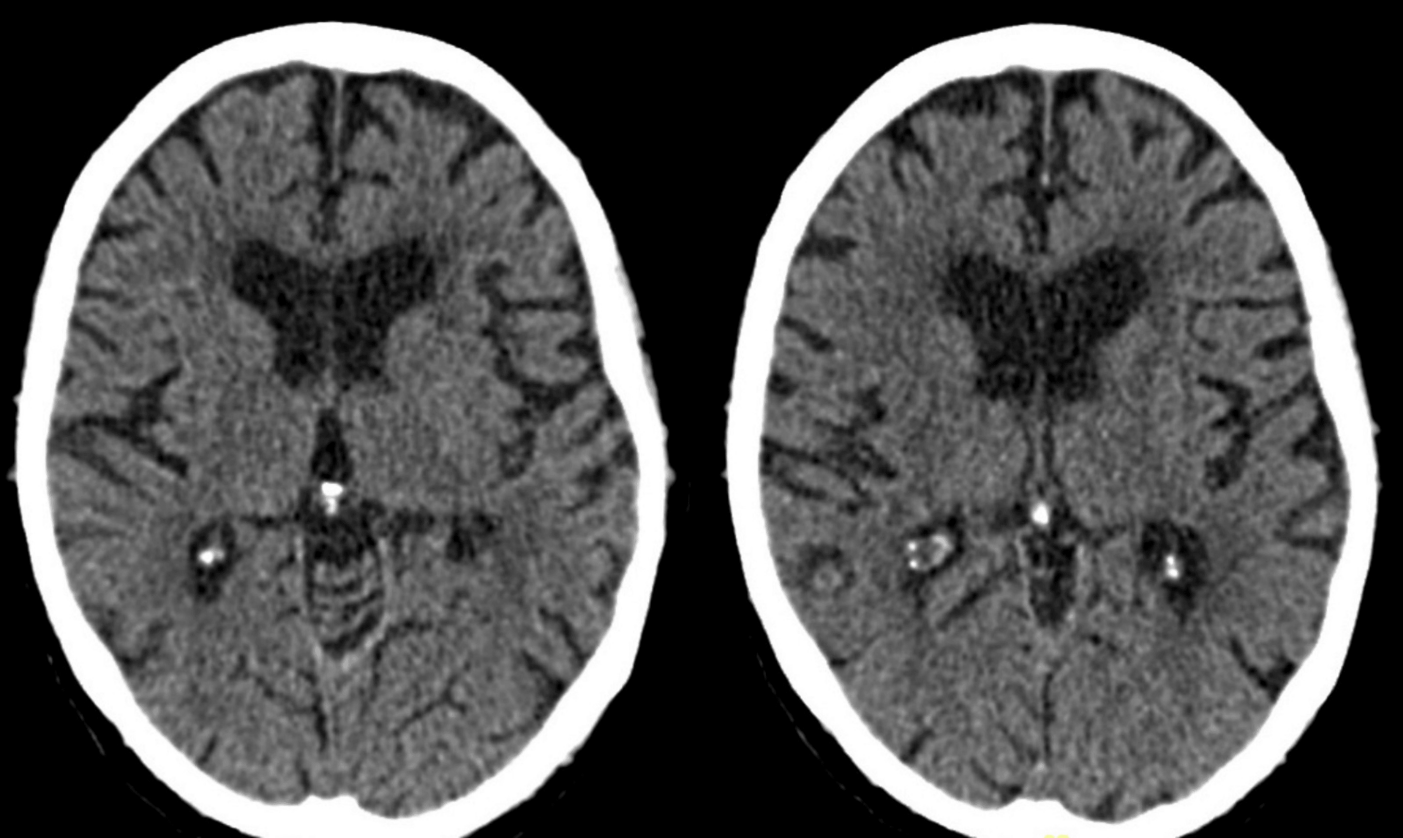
Non-contrast computed tomography (NCCT) head that shows no evidence of acute infarction in bilateral thalami.
